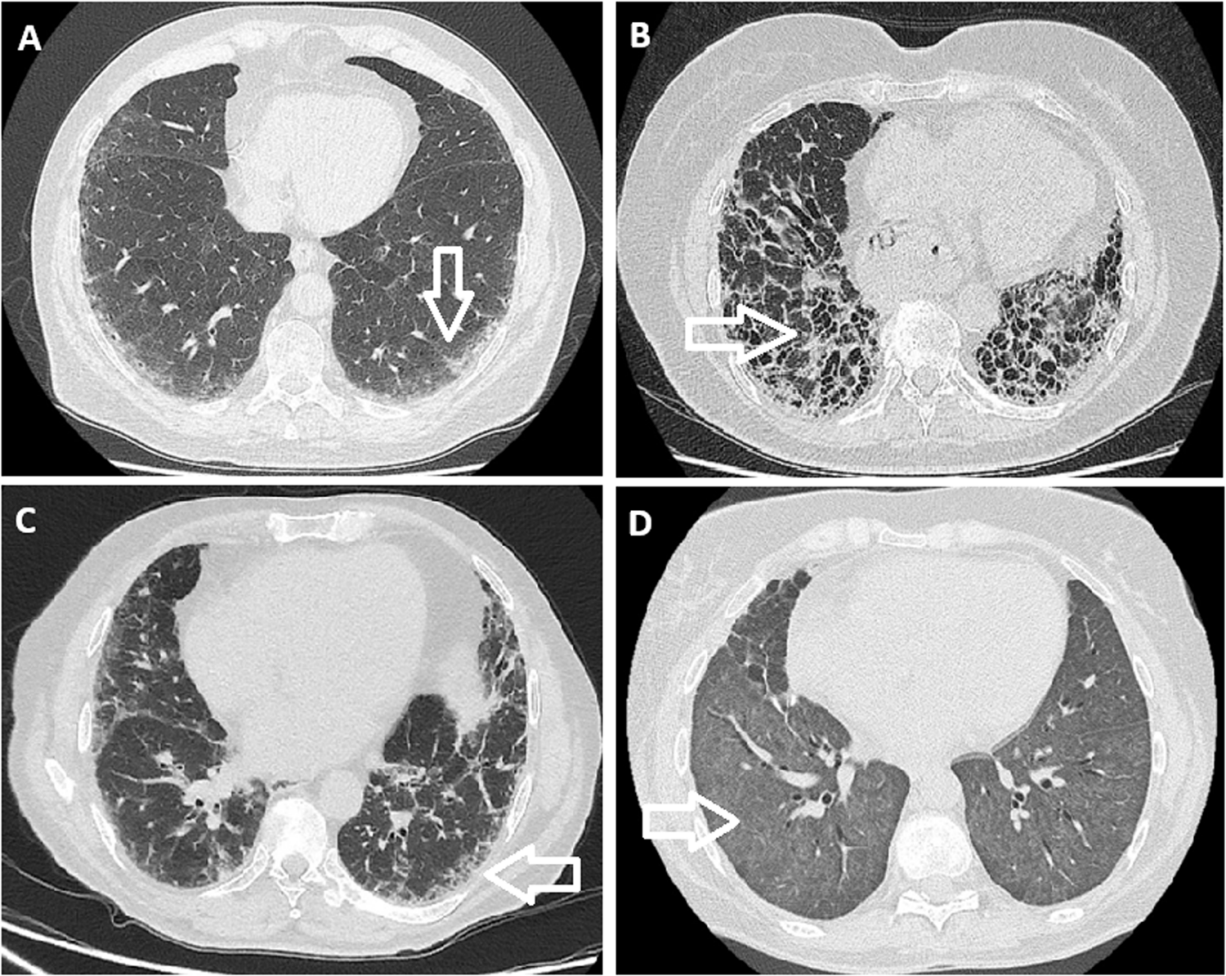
Example HRCT images of ILD in patients with anti‐SRP antibody. (A) Subpleural opacities in both lower lobes suggestive of NSIP pattern of ILD. (B) Bilateral peripheral reticulations, traction bronchiectasis, pleural‐parenchymal interface distortion and honeycombing, in keeping with UIP pattern of pulmonary fibrosis. (C) Fibrotic lung disease more in keeping with NSIP/OP than UIP. (D) Extensive bilateral ground‐glass opacification with interlobular septal thickening in keeping with pulmonary haemorrhage.
